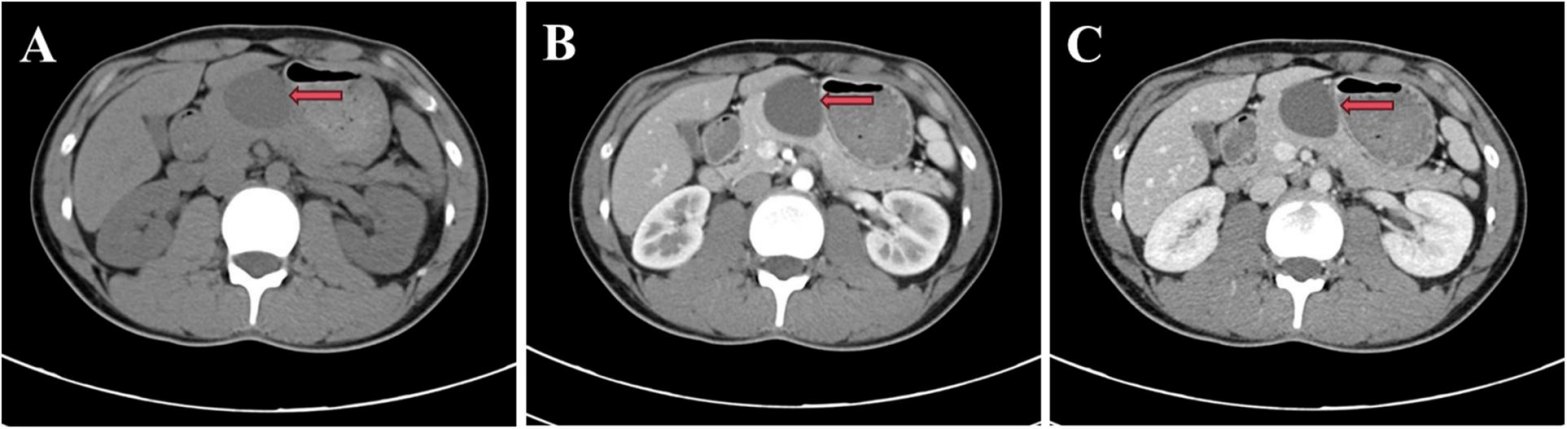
The lesion in the pancreatic body was detected on CT scan, which was marked by red arrows. (A, plain scan; B, arterial phase; C, venous phase).
